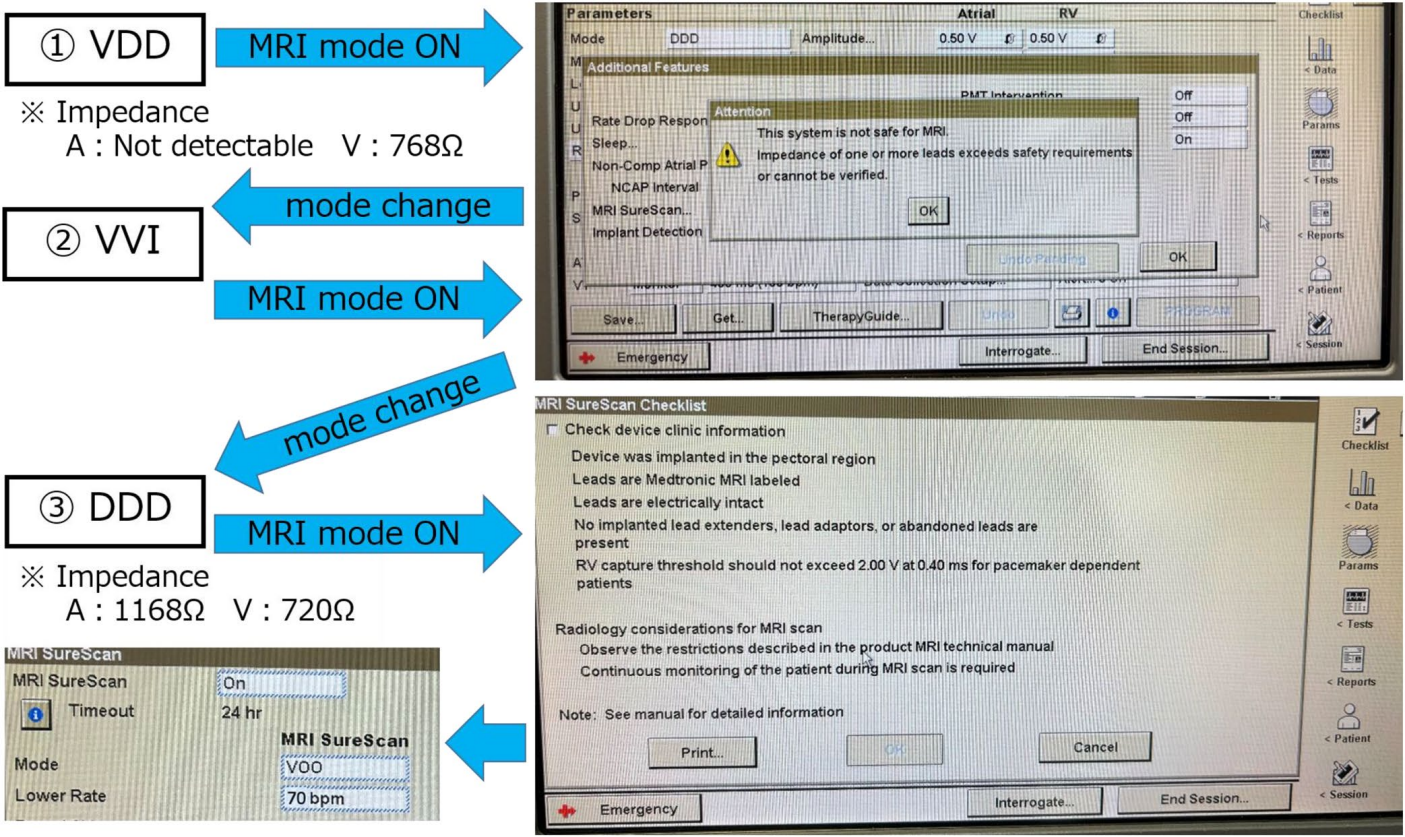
Steps to enter MRI Mode. Although switching to MRI mode from VDD mode or VVI mode was not possible, it became possible after switching to DDD mode.
